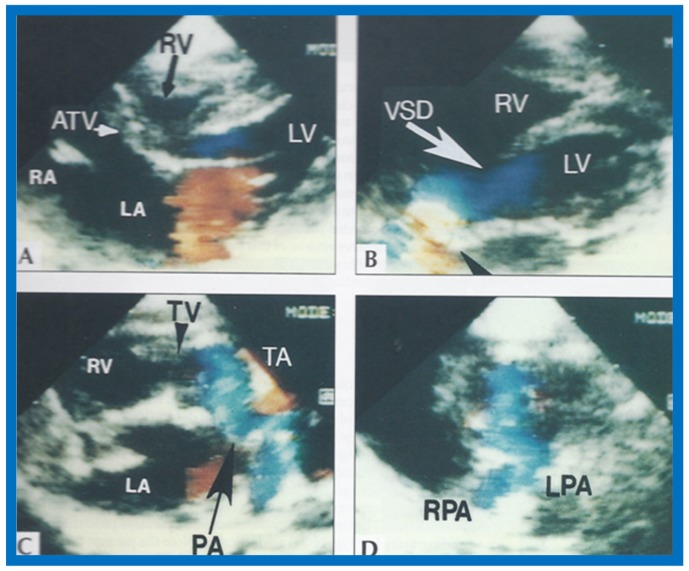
Video frame from a two-dimensional echocardiographic and color Doppler study demonstrating (A) atretic tricuspid valve (ATV) between the right atrium (RA) and right ventricle (RV) and blood flow from the left atrium (LA) into the left ventricle (LV) across the mitral valve. The RV (arrow) is very small and hypoplastic. (B) LV and RV with a large ventricular septal defect (VSD) below the truncus arteriosus (TA). Turbulent flow across the truncal valve suggests truncal valve stenosis. (C) origin of the pulmonary artery (PA) from the TA by color flow (arrow), and (D) division of right (RPA) and left (LPA) pulmonary arteries from the PA (labeled in d) in a short-axis view. TV, truncal valve leaflets. Reproduced from Rao P.S., et al. [22].
